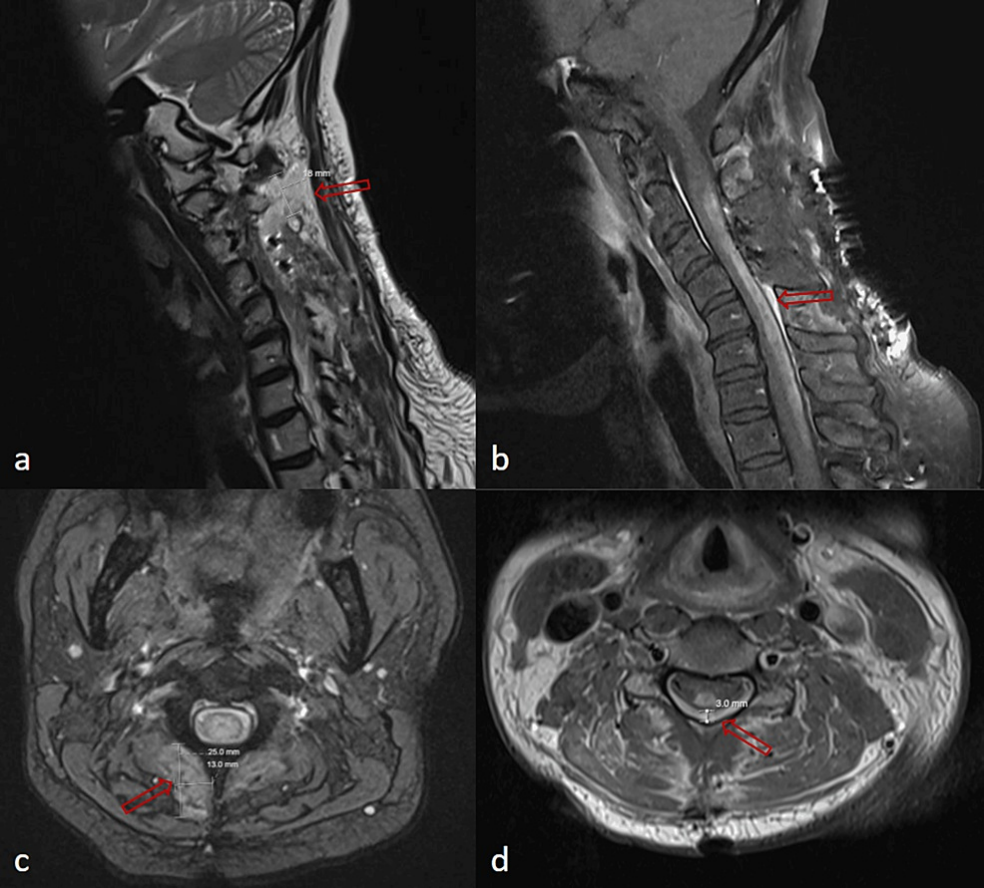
Sagittal (a and b) and axial (c and d) T2 (a and c) and T1 fat-saturated (b and d) weighted magnetic resonance images. Extensive postoperative edema and enhancement throughout the soft tissues including a 2.5 x 1.3 x 1.8 cm collection at the right paraspinal musculature of C2-4. (a and c, red arrow). Additionally, there is a hyperintense, enhancing, dorsal epidural collection measuring up to 3 mm in AP dimension and extends from C4-T1 (b and d, red arrow), possibly reflecting an epidural abscess.
